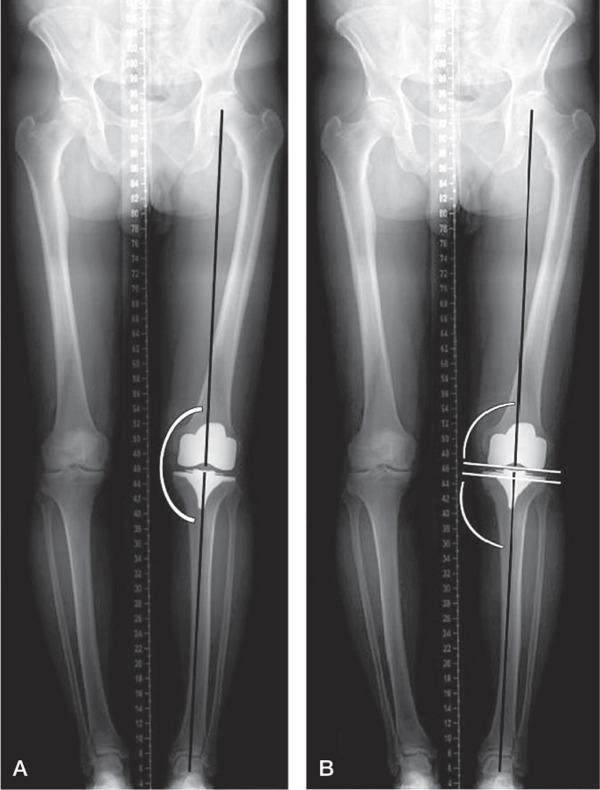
A. long-leg radiograph. Mechanical axis of the leg measured on a standing long-leg radiograph as the medial angle between the mechanical axis of the femur, defined as the line between the center of the hip and the center of the femoral component, and the mechanical axis of the tibia, defined as the line between the center of the tibial component and the center of the ankle. B. Long-leg radiograph. FFC and FTC angles were measured as the medial angles between the femoral component and the mechanical axis of the femur and between the tibial component and the mechanical axis of the tibia, respectively.
